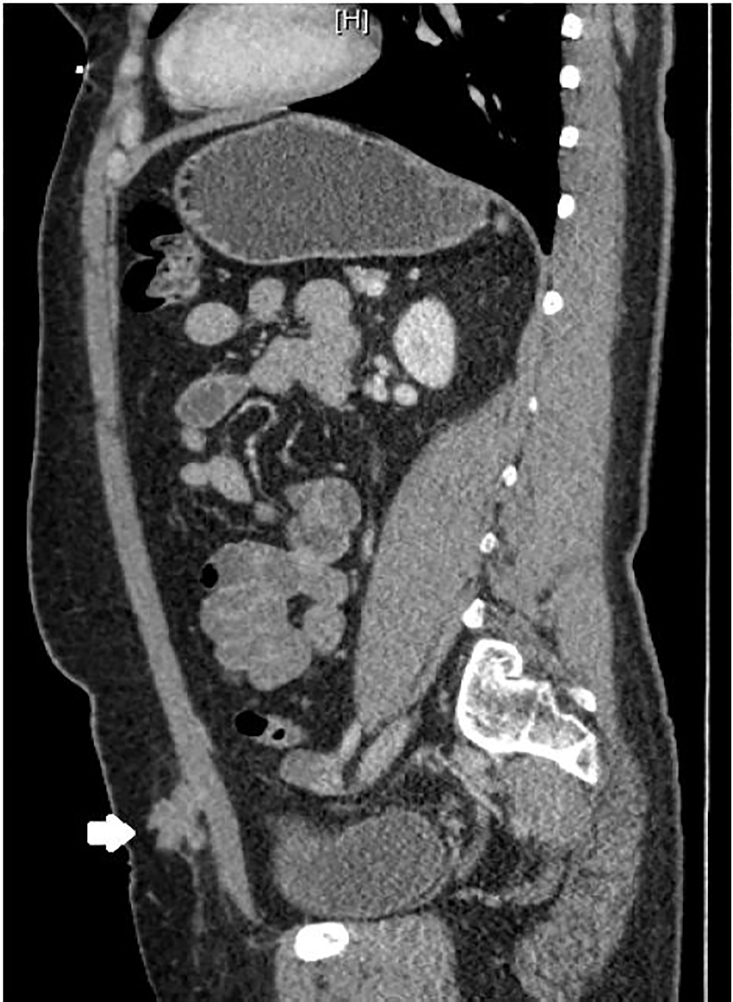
Computed tomography abdomen of the mass in sagittal plane demonstrating enhancing soft-tissue mass with irregular margins (arrow).
